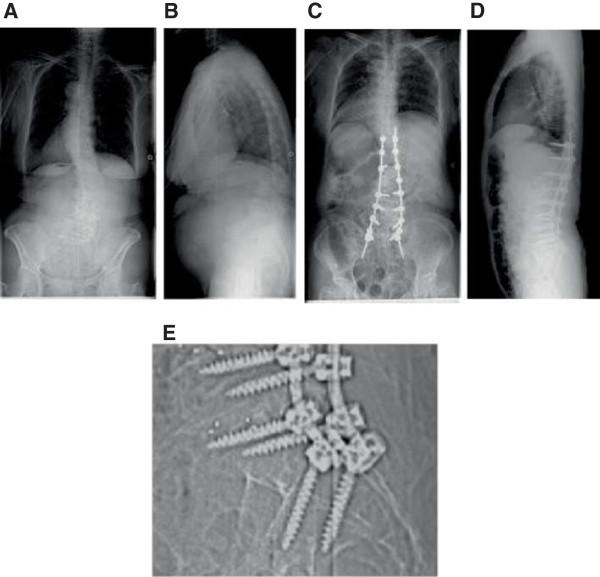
Radiographs of a 77-year-old female with degenerative kyphoscoliosis. A: Posteroanterior view of the preoperative whole spine. B: Lateral view of the preoperative whole spine. C: Posteroanterior view of the postoperative whole spine. D: Lateral view of the postoperative whole spine. E: Intrasacral screws are seen in the magnified view of the lumbosacral junction.
